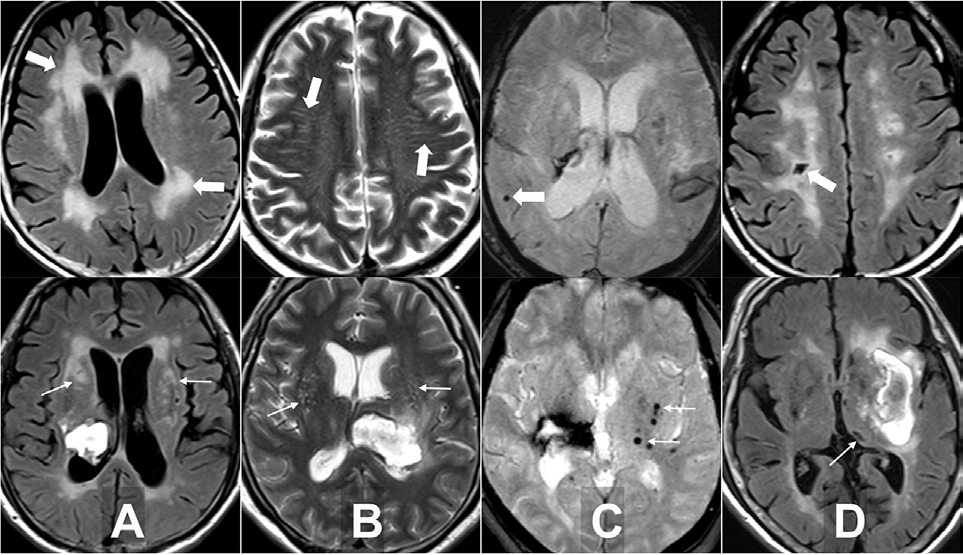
Examples of the four magnetic resonance imaging markers of small vessel disease. (A) White matter hyperintensity is a signal abnormality in the white matter that shows hyperintensity on fluid-attenuated inversion recovery (FLAIR) and without cavitation, it is categorized into periventricular (thick arrows) and deep (arrows). (B) Enlarged perivascular space is a fluid-filled space that follows the typical course of a vessel and is hyperintensity on T2WI. It is categorized into centrum semiovale (thick arrows) and basal ganglia (arrows). (C) Cerebral microbleed is a small, generally between 2 and 10 mm round lesion of signal void with associated blooming seen on T2*-weighted gradient echo images and is categorized into lobar (thick arrow) and deep (arrows). (D) Lacuna is a round or ovoid fluid-filled cavity on FLAIR of between 3 and 15 mm in diameter, consistent with previous small subcortical infarct and is categorized into lobar (thick arrow) and deep (arrow).
